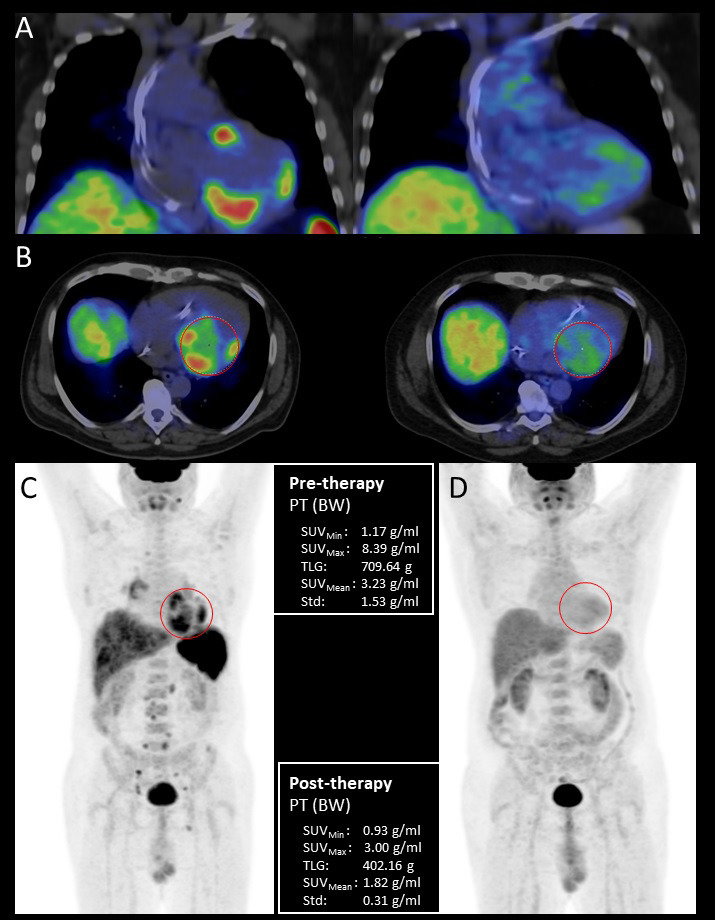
Pre- and posttreatment FDG-PET/CT examination of a 44-year-old
